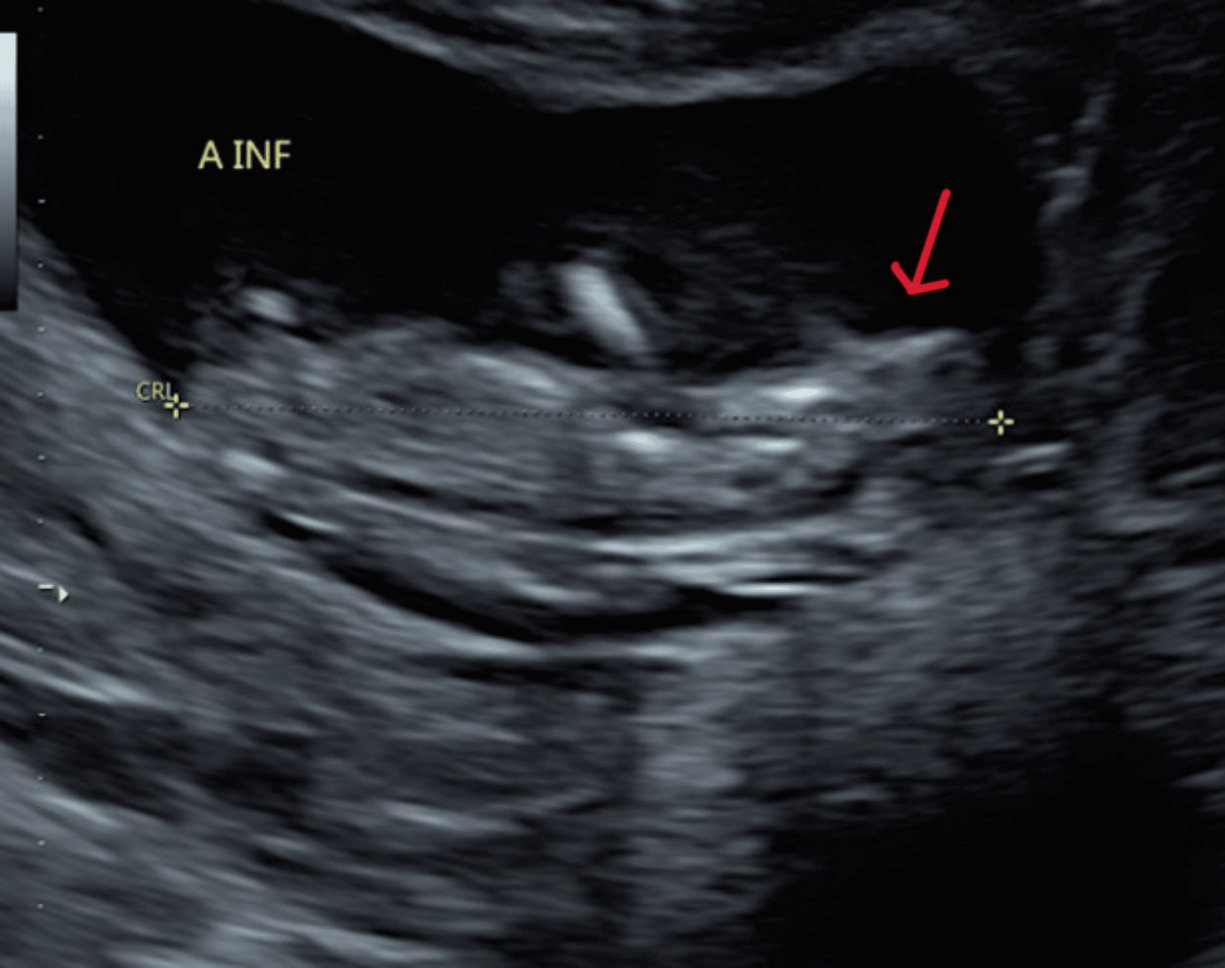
Ultrasound of twin A at 13.1 weeks gestation demonstrating anencephaly Red arrow is pointing to the cranium.
